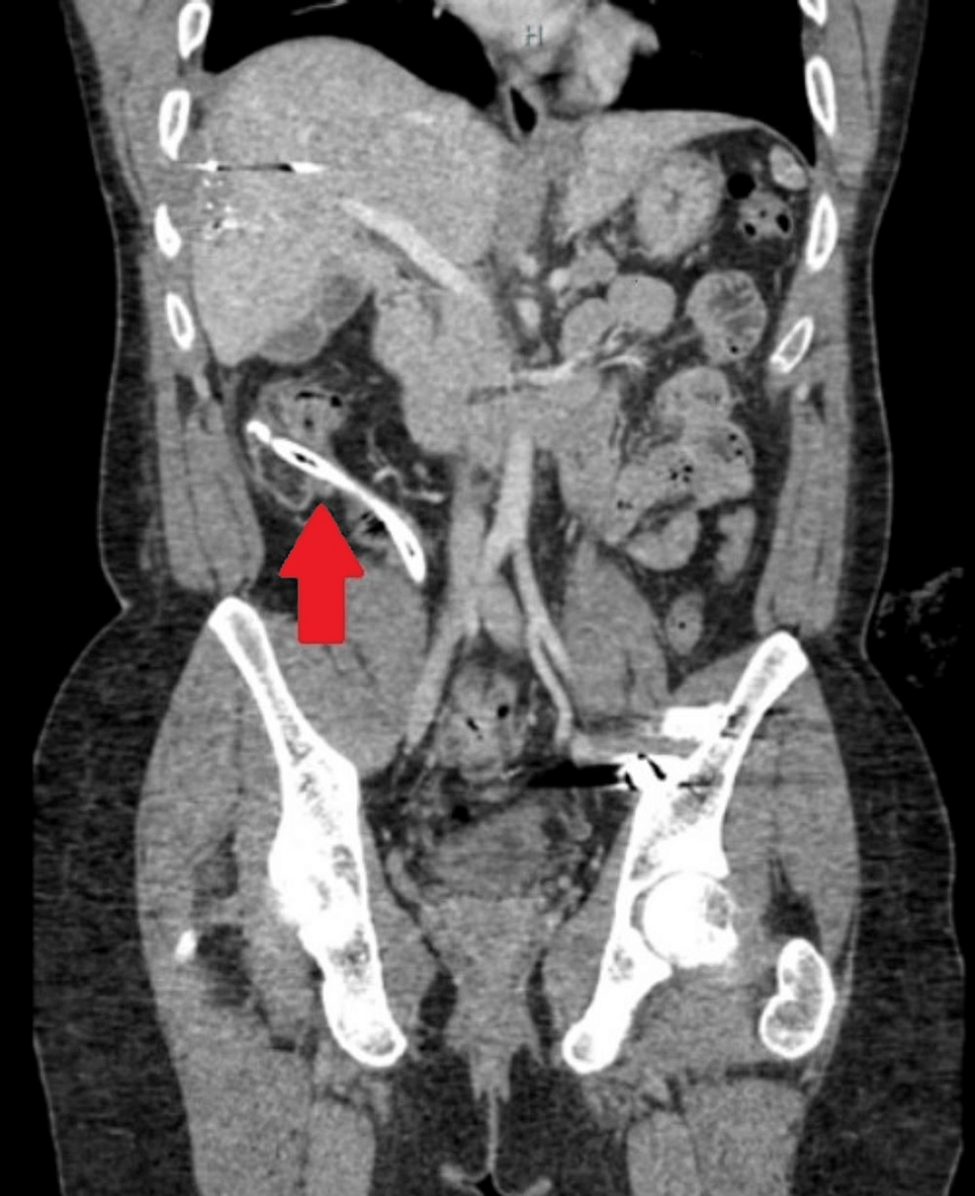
CT imaging with arrow indicating retained Malecot catheter
